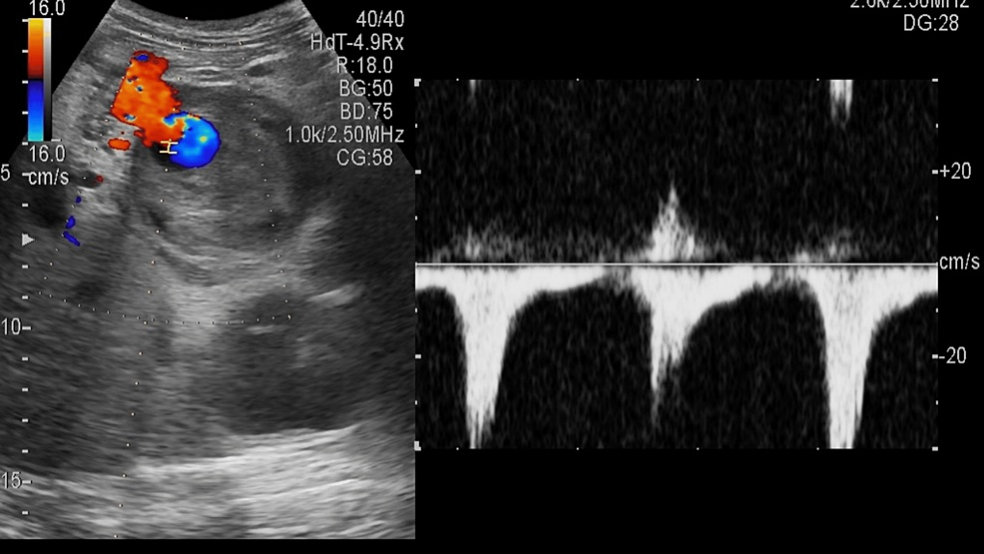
Pulsed Doppler of the affected artery showing to and fro waveform characteristic of pseudoaneurysm.
